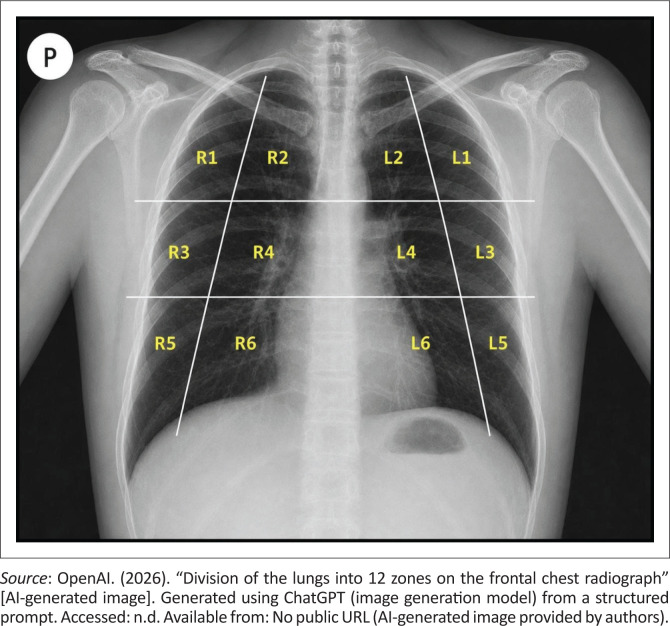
Division of the lungs into 12 zones on the frontal chest radiograph.
